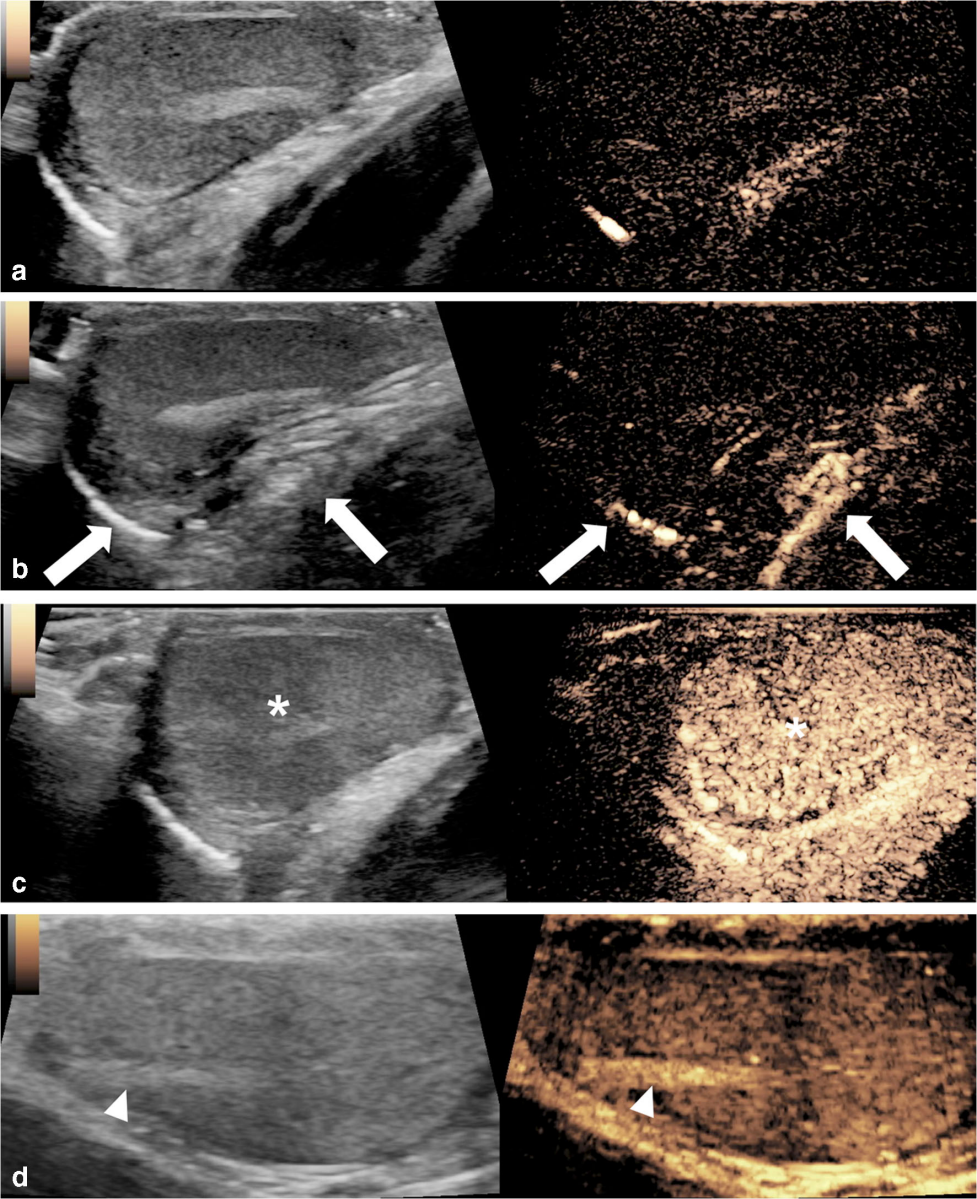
Advanced puberty in an 11-year-old boy undergoing high-resolution contrast-enhanced ultrasound (CEUS) of the right testis to assess for tumor. Normal testicular perfusion. a–d Transverse CEUS image with dual display of contrast (*right*) and gray-scale (*left*) modes. Two seconds post contrast injection (a), no intratesticular enhancement is visible. Eleven seconds post contrast injection (b), the first microbubbles appear in the scrotum and the testicular tunica (*arrows*). Twenty-two seconds post-contrast injection (c), there is homogeneous enhancement of the testicular tissue (*asterisk*). One hundred twenty seconds post-contrast injection (d), there is homogeneous enhancement of the testicular tissue including the mediastinum testis (*arrowhead*), without pathological changes
